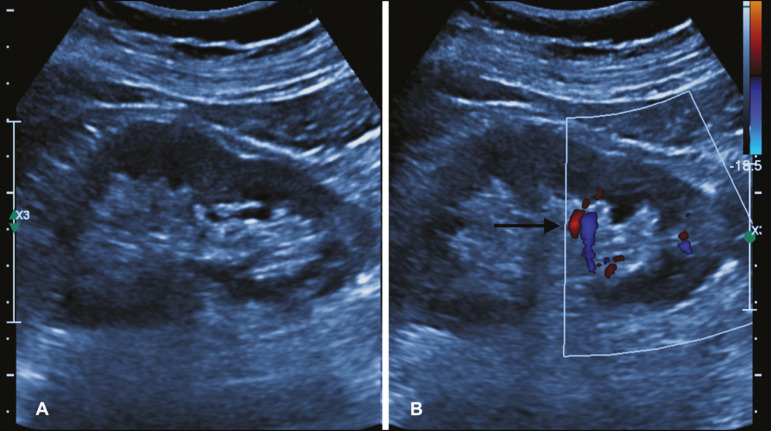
A: Follow-up ultrasound performed 60 min after the biopsy, showing no sign of free fluid surrounding the left kidney. B: Ultrasound with color Doppler showing normal renal vascularization without signs of bleeding (arrow).
